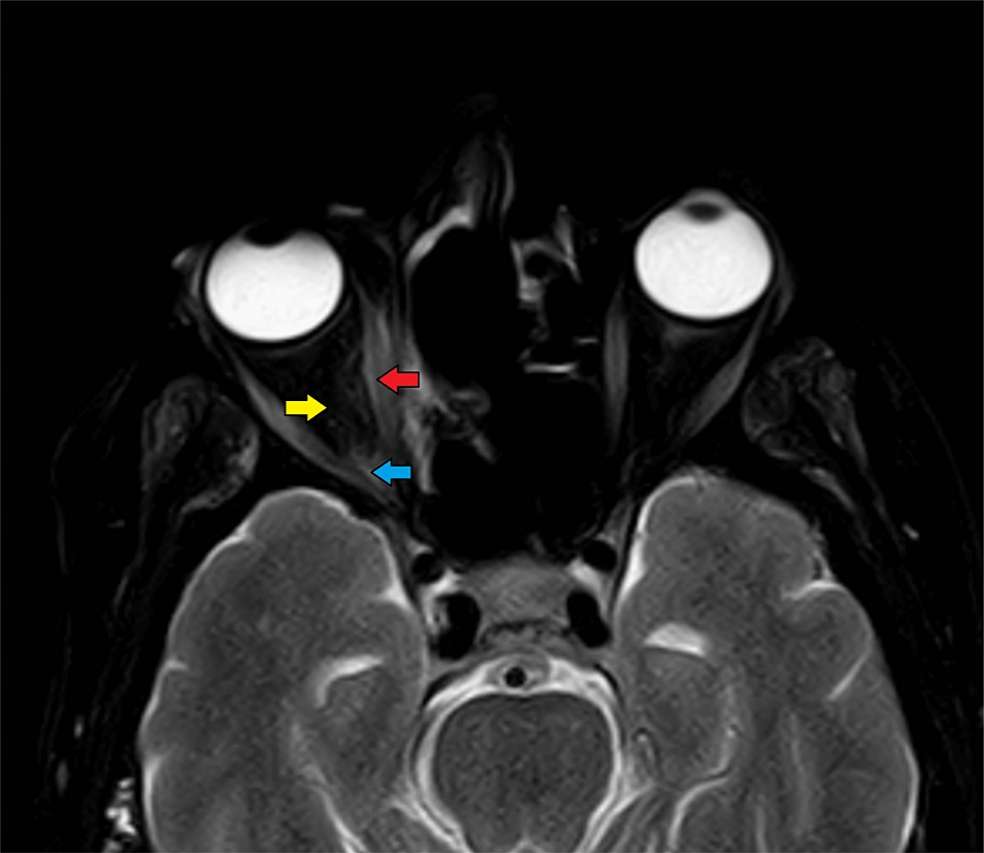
Magnetic resonance imaging (T2 fat-saturated axial section) showing retro-orbital fat stranding (yellow arrow), slightly bulky right medial rectus muscle (red arrow), and the extension of the disease process into the orbital apex (blue arrow).
